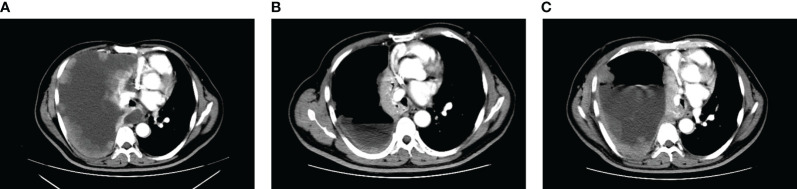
CT images corresponding to the lung lesions in patient before oral Lorlatinib (A), significant remission of pleural lesions and pleural effusion during oral Lorlatinib (B), and pleural lesions and pleural effusion that developed after 5 months of oral Lorlatinib (C).
